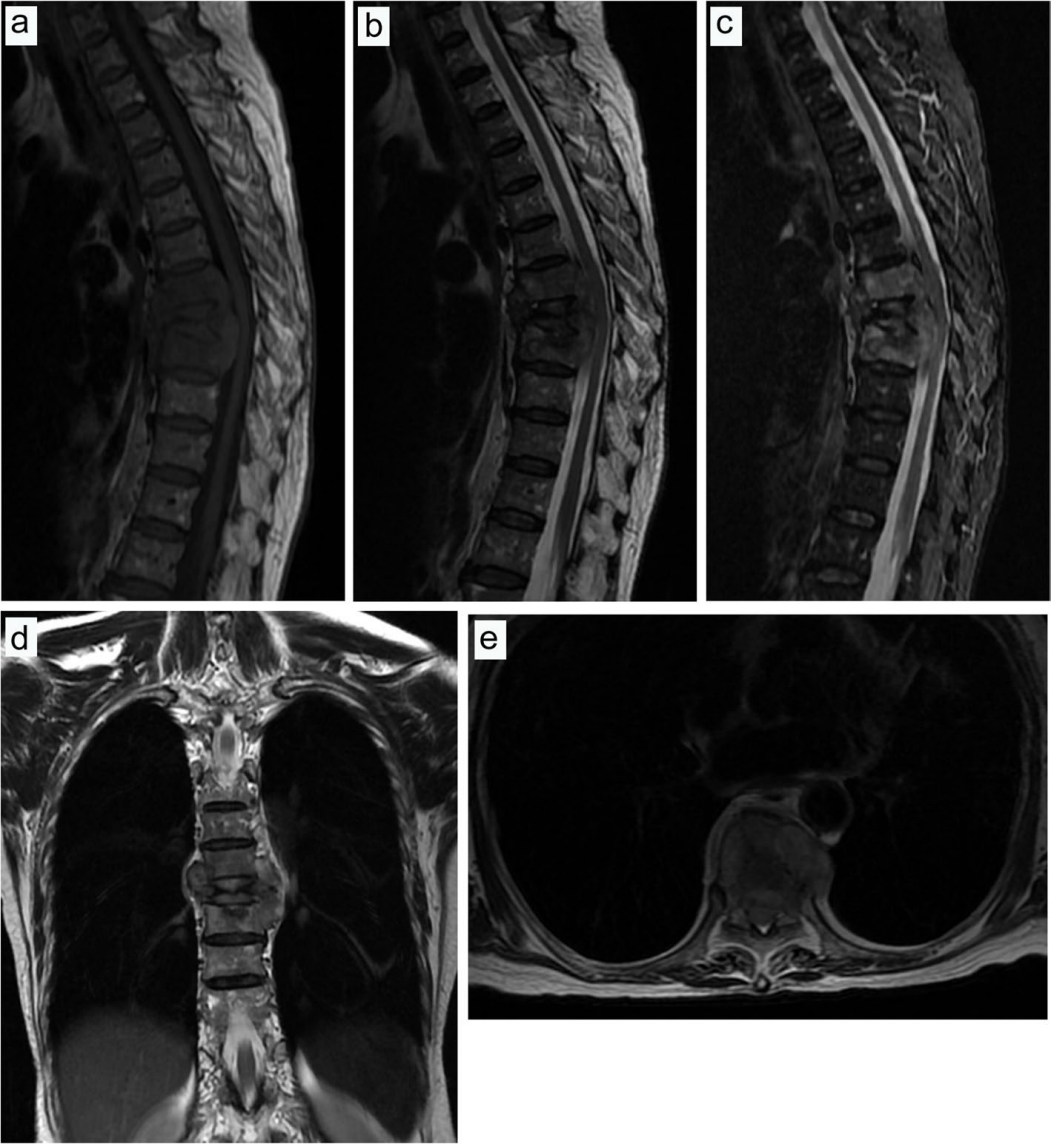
Preoperative MRI examination in one patient with intraoperative blood transfusion. a, MRI examination in the sagittal T1 sequence. b, MRI examination in the sagittal T2 sequence. c, MRI examination of T2 lipid compression sequence in sagittal position. d, MRI examination in the coronal T2 sequence. e, MRI examination of the T2 sequence in cross-section
